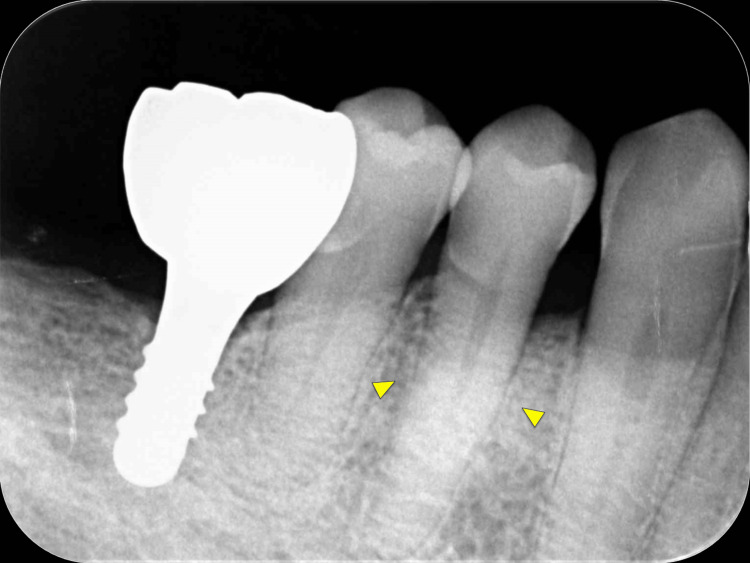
Preoperative intraoral radiographic image. It is difficult to evaluate the condition of the alveolar bone defect around tooth #44 on the intraoral radiographic image (arrowheads).
